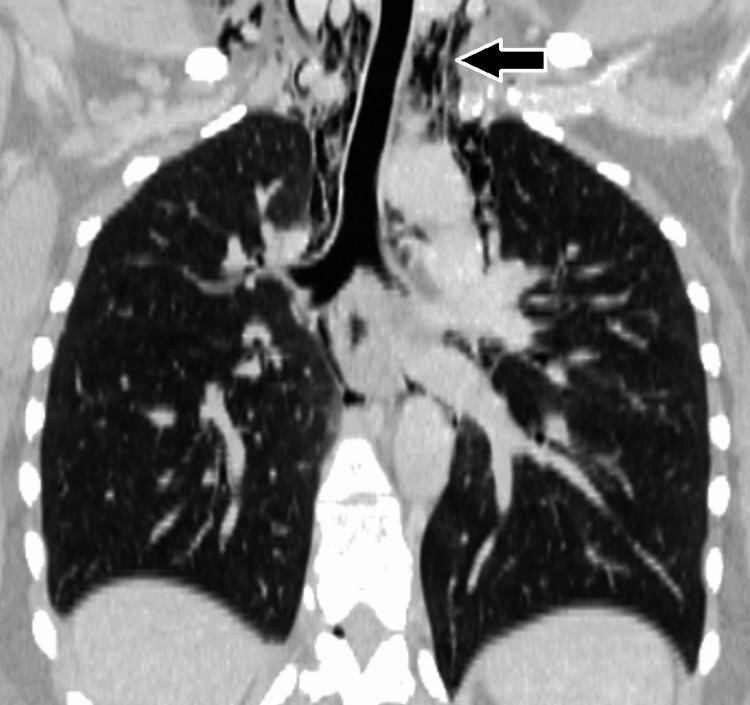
Coronal reformatted computed tomography image of the chest confirming the presence of extraluminal air (arrow) within the superior mediastinum, adjacent to the trachea
